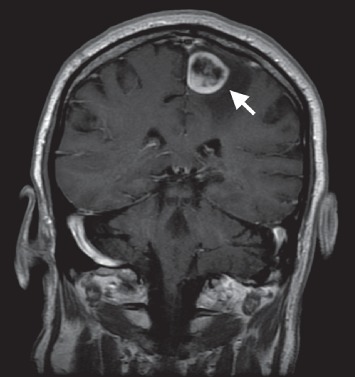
T1 postgadolinium MRI brain with coronal view. This reveals a peripherally enhancing and centrally necrotic appearing mass in the left frontoparietal lobe with surrounding edema.
